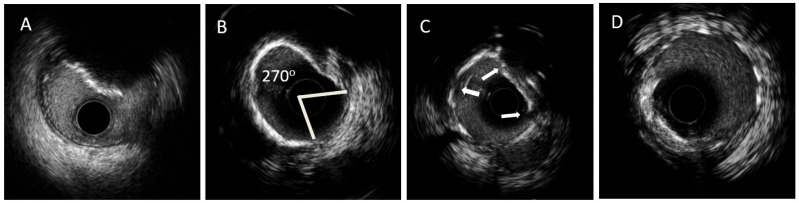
IVUS images during PTCA of calcified lesions. Legend: (A). Calcified nodule. (Β). Concentric calcium of 270 degrees. (C). Presence of calcium fractures after IVL treatment (white arrows). (D). IVUS after stent implantation. Abbreviations. IVUS: IntraVascular UltraSound, PTCA: Percutaneous Transluminal Coronary Angioplasty.
